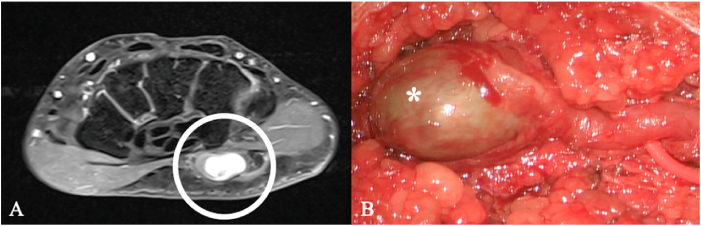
Thrombosed Aneurysm in a 47-year-old man, initially suspected to be an ulnar-nerve schwannoma: (A) Axial fat-suppressed T2-weighted MRI shows a well-circumscribed subcutaneous mass at the ulnar neurovascular bundle (white circle), but (B) intraoperatively it proved to be a thrombosed ulnar artery aneurysm (∗), which was completely excised with preservation of both nerve and vessel.
